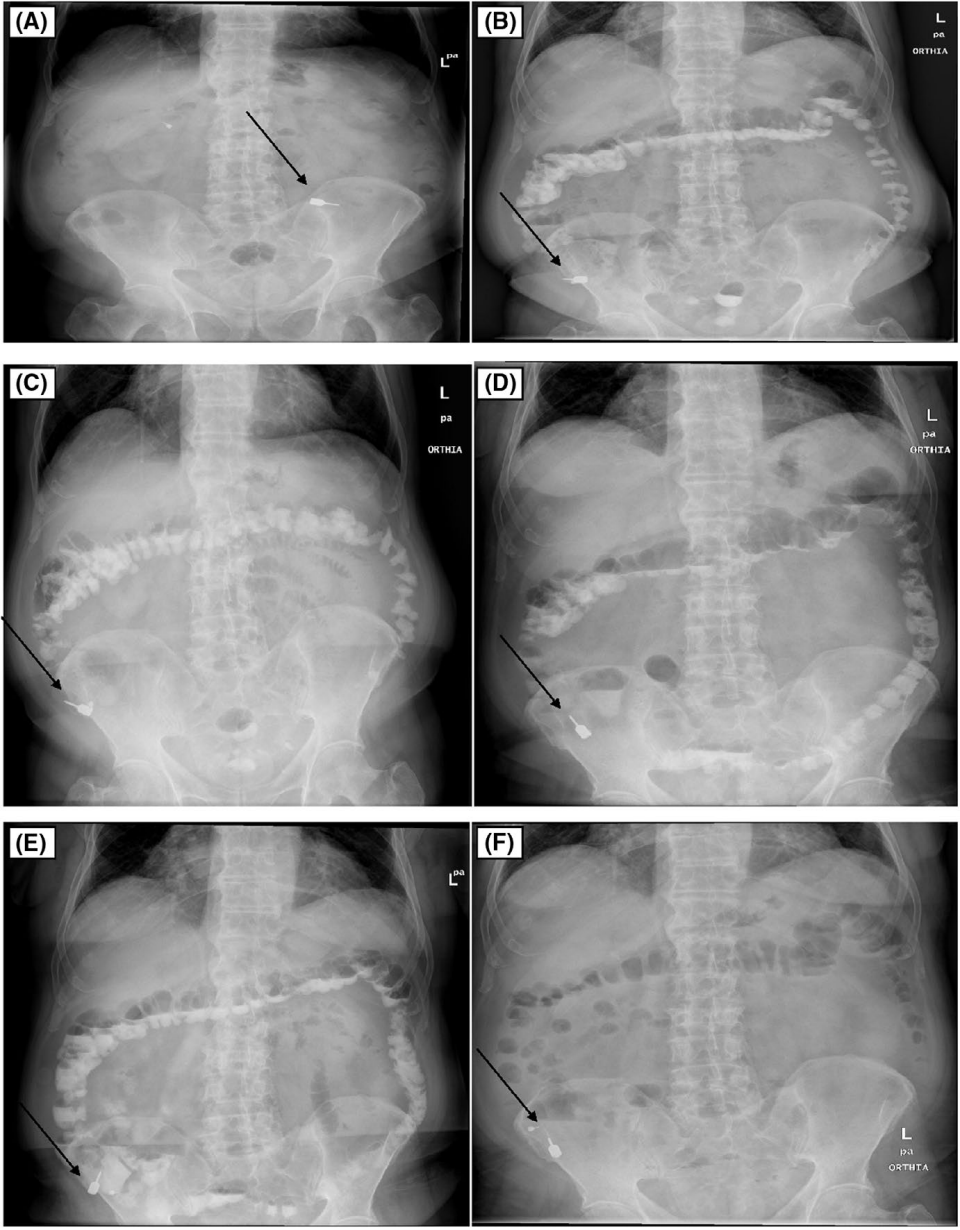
Abdominal radiography: showed a hyperdense, foreign body in the left lower quadrant 2 hours after ingestion (A). Movement of foreign body in the right lower quadrant 24 hours after ingestion (B). The foreign body remains in the same position 48 hours (C), 72 hours (D), 5 days (E), and 7 days (F) after ingestion
